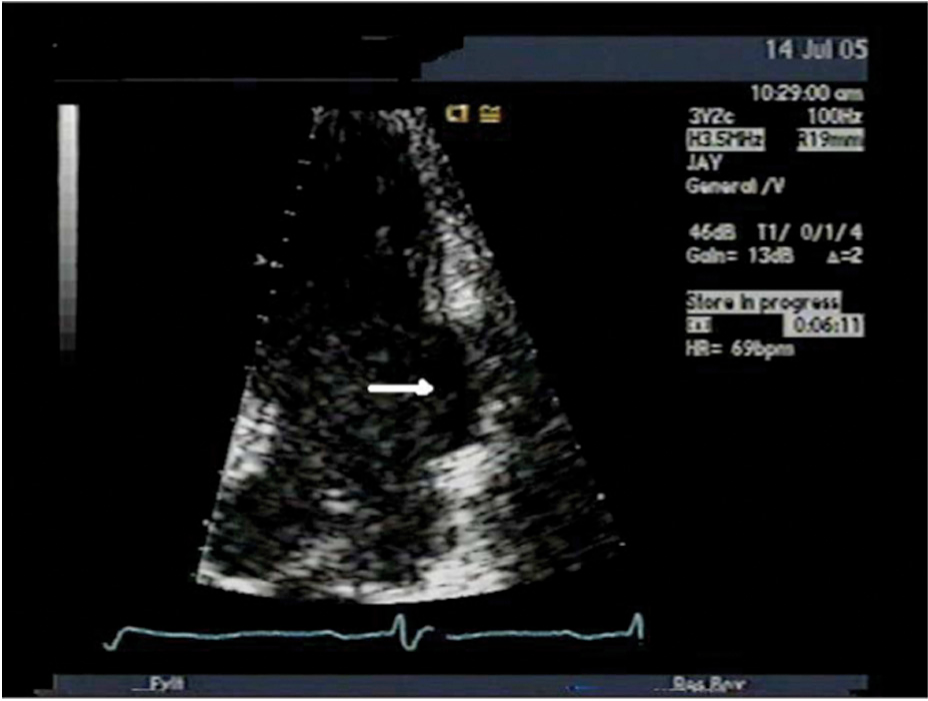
Transthoracic echocardiogram done 10 years after patch repair of the subacute ventricular rupture (arrow).
